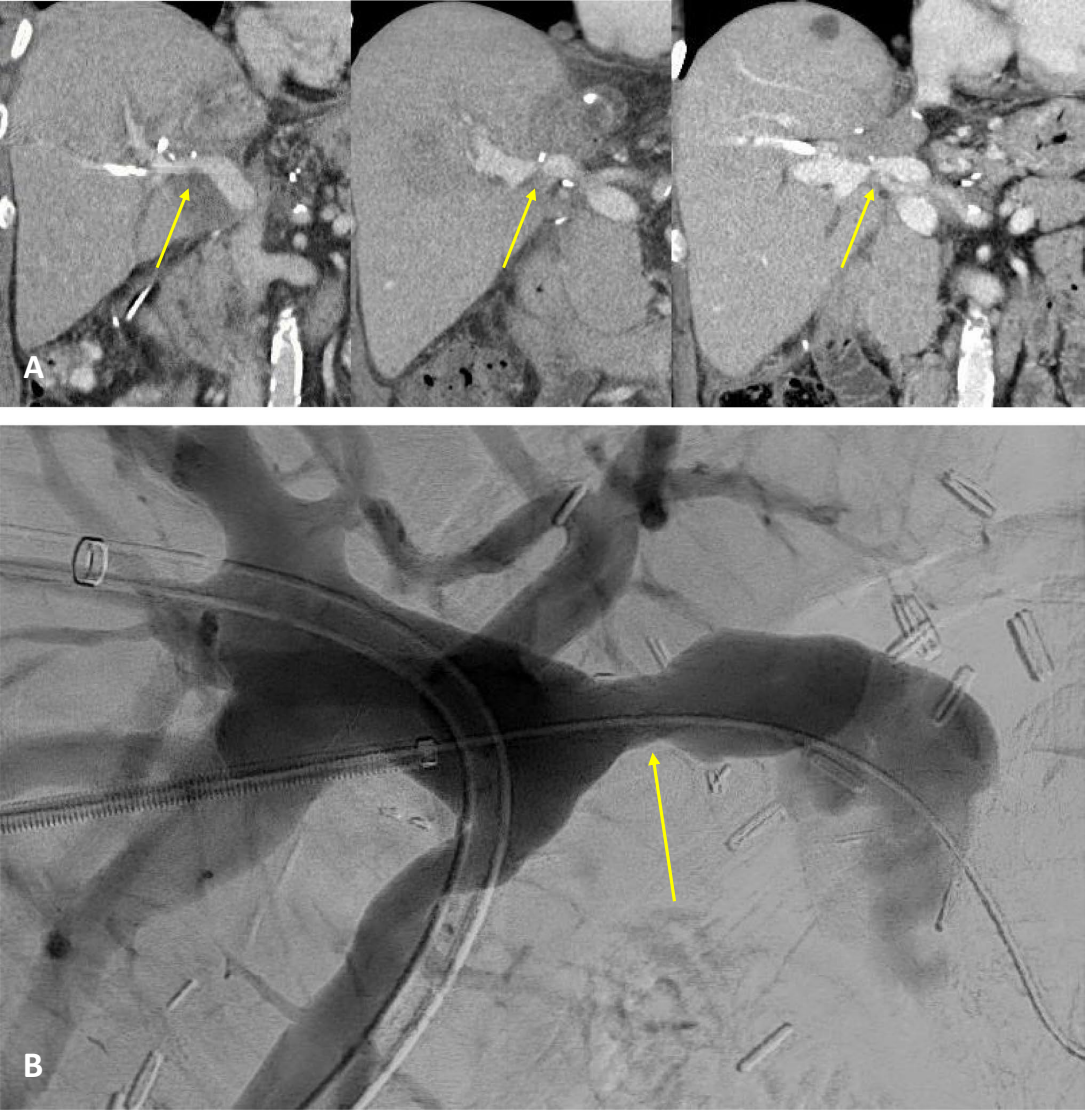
Complications after PPP reconstruction. A CT scan of the PV after PPP reconstruction during left hemihepatectomy for CCC with progressive vascular stenosis at 8 days and 2 and 15 months postoperatively; B percutaneous transluminal angioplasty (PTA) with stent (arro implementation of PV at 15 months postoperatively)
